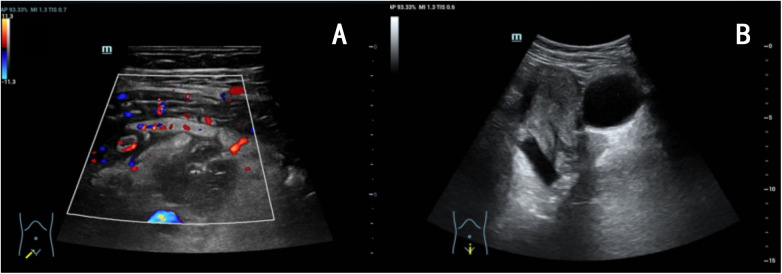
Examinations before nendoscopic retrograde appendicitis therapy. (A) Transabdominal ultrasonography demonstrated an appendiceal abscess in the right lower quadrant, measuring approximately 41 × 25 × 29 mm, intralesional blood flow signals are identified. (B) Transabdominal ultrasonography demonstrated an endometrial thickness of 9 mm with no definitive gestational sac identified within the uterine cavity.
